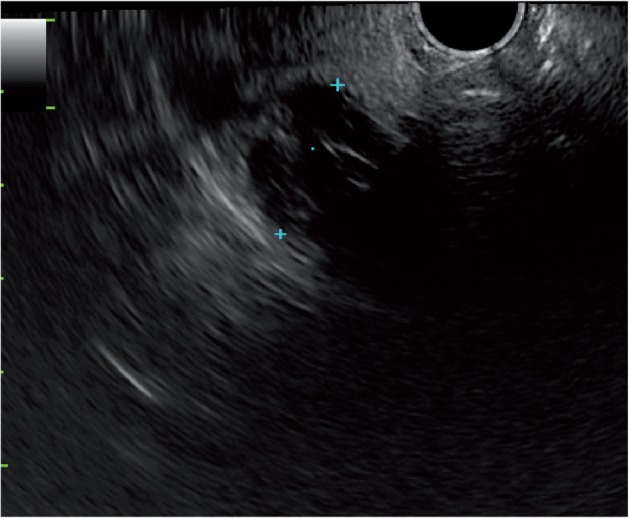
Incidental endoscopic ultrasound finding of a 17 mm multi-locular cystic lesion of the thyroid. The endoscope is advanced by 18–20 cm and is orientated towards the anterior wall of the oesophagus.
